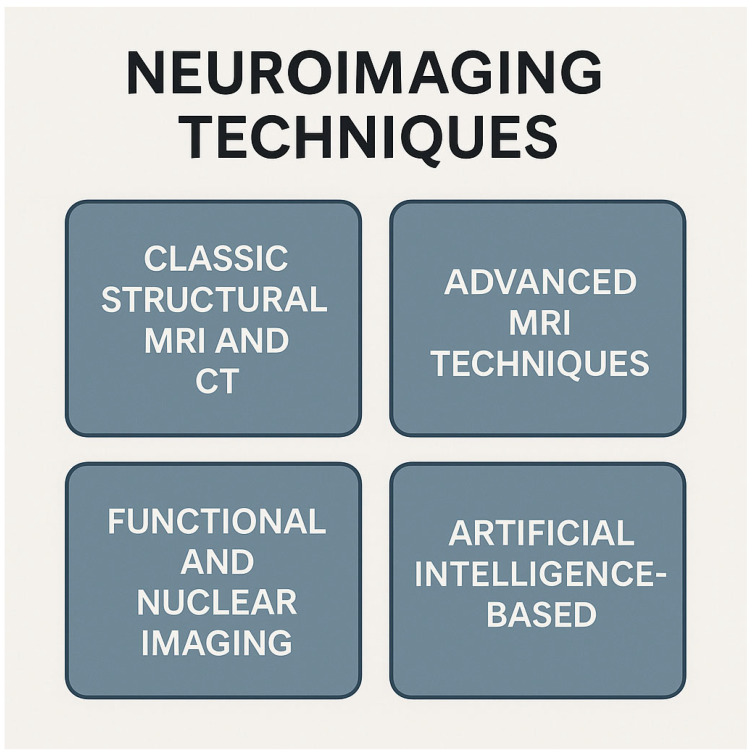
Classification of neuroimaging techniques into four categories. Conventional structural MRI and CT provide detailed anatomic images; advanced MRI methods offer microstructural and hemodynamic insights; functional and nuclear imaging map brain activity, metabolism, and molecular targets; and artificial intelligence-based approaches are used for image analysis, lesion detection, and predictive modeling. Abbreviations: CT—computer tomography; MRI—magnetic resonance imaging. Created with Biorender.com.
